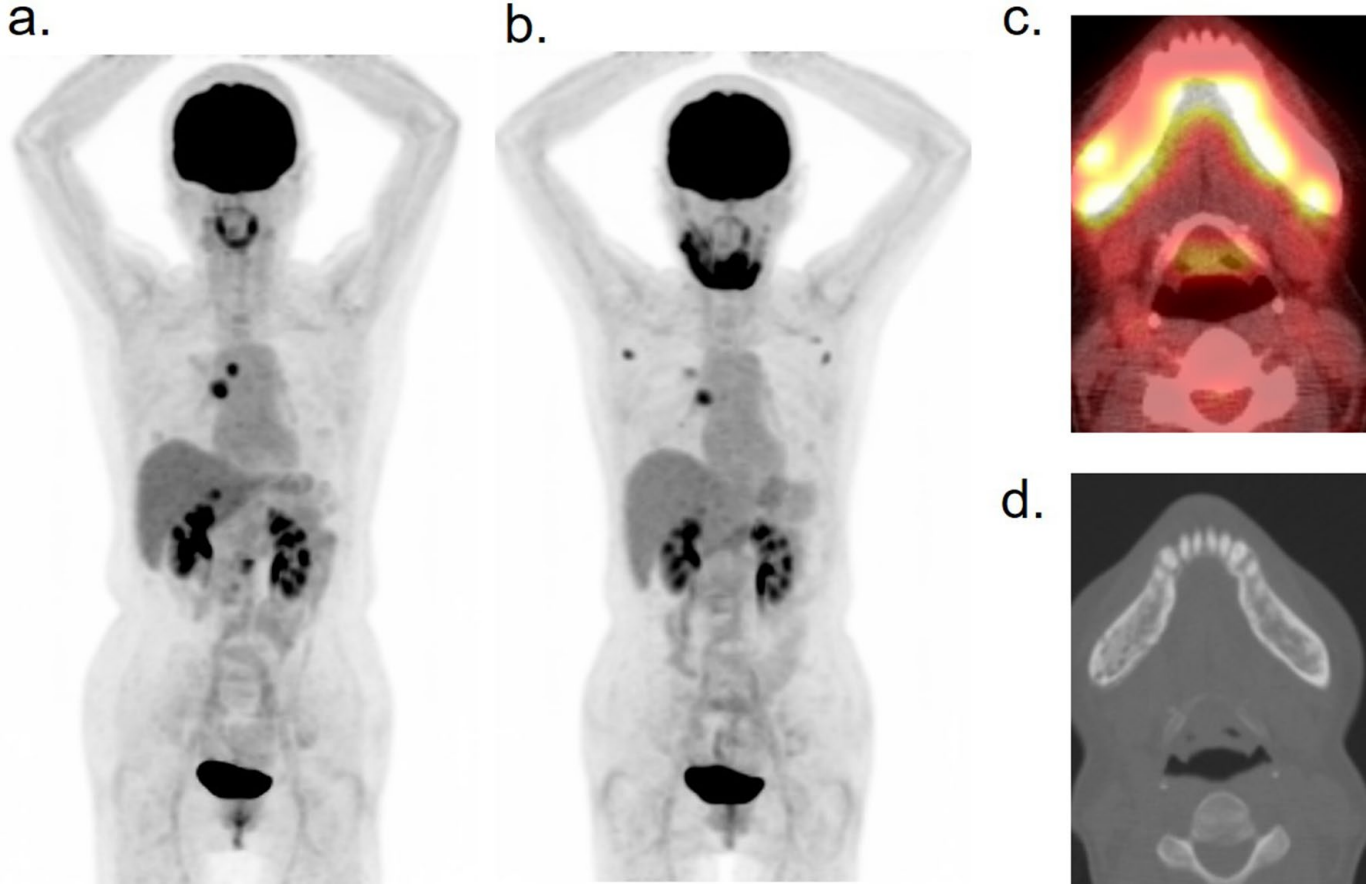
Patient with lung cancer with bone metastasis who developed medication-related osteonecrosis of the jaw due to bisphosphonate. The first PET scan (a) revealed metastatic lesions in the mediastinum, right adrenal gland, and a lumbar vertebra. The second PET scan detected the abnormality as an intense accumulation in the mandibular bone (b, c) earlier than the CT (d)
